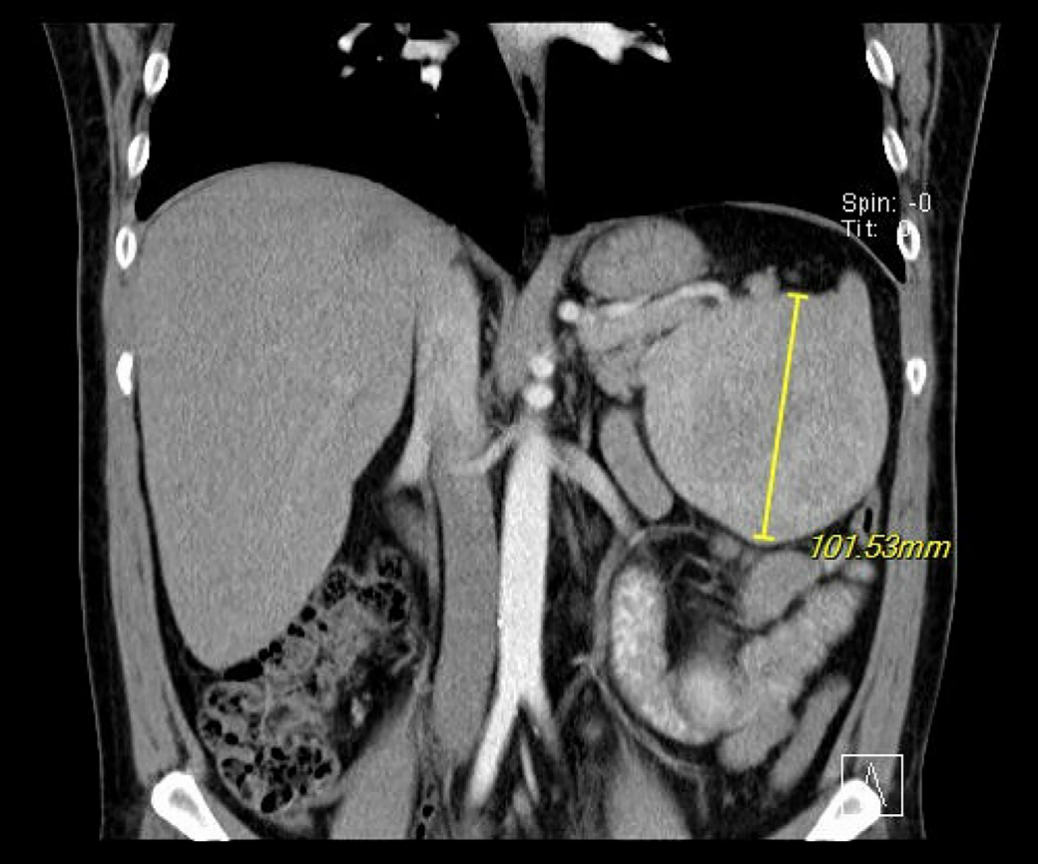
Coronal view CT w/ contrast of splenic mass.
